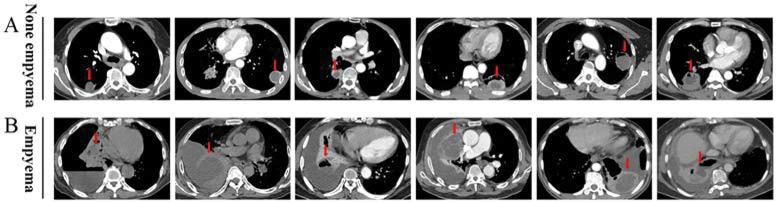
The chest CT image characteristics of pulmonary abscesses in patients without or with empyema A, Representative images from 76 cases of pulmonary abscess without empyema. B, Representative images from 25 cases of pulmonary abscess-related empyema. The arrow indicates a pulmonary abscess.
